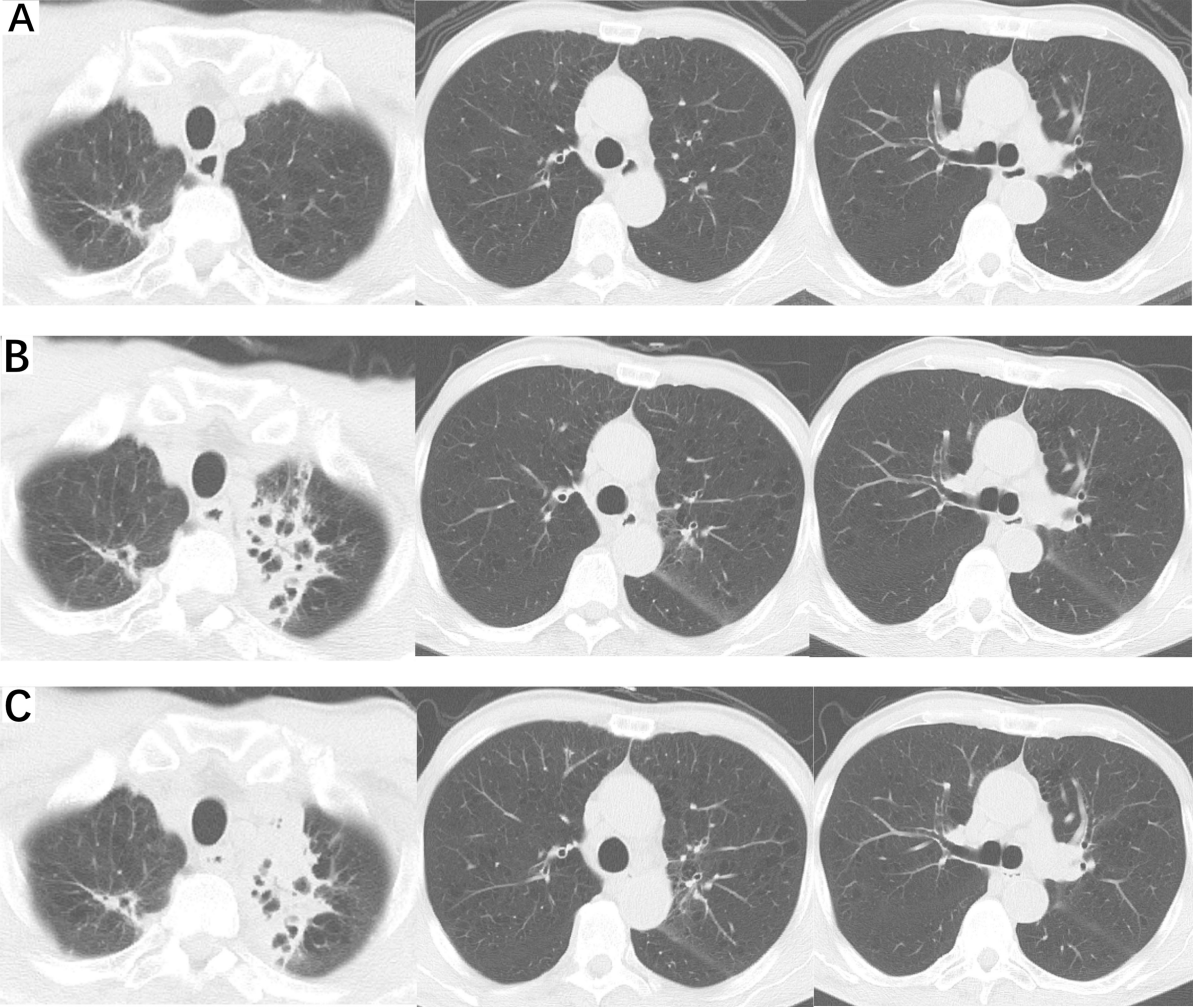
Chest computed tomography (CT) on March 12, 2024 showed roughly normal lungs (A). Chest CT on June 12, 2024 revealed patchy opacities with cavitation in the left lung (B). Chest CT on June 21, 2024 showed the lesion was not absorbed after 7 days of antibiotic therapy (cefoperazone‐sulbactam plus doxycycline) (C).
